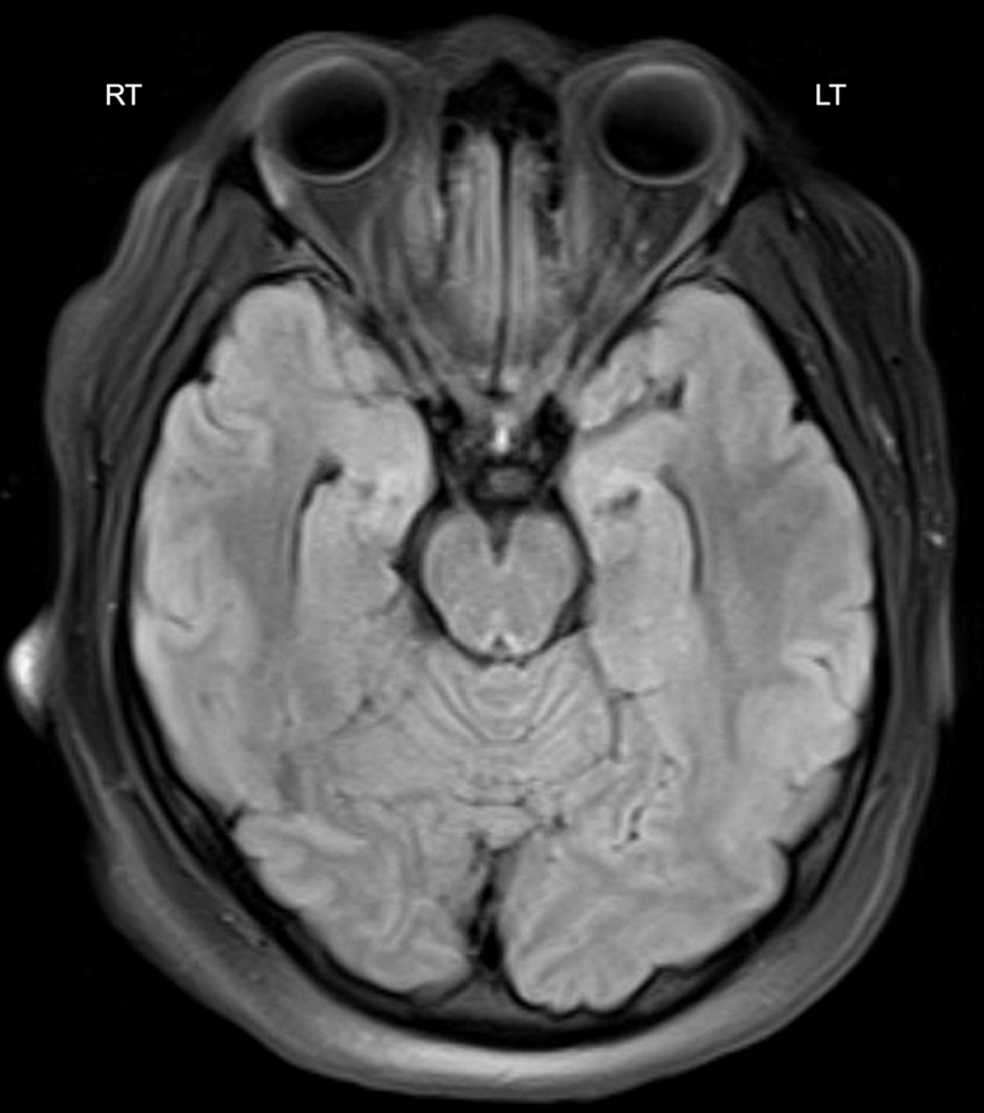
Normal axial flair T2-weighted MRI at the level of inferior colliculus
